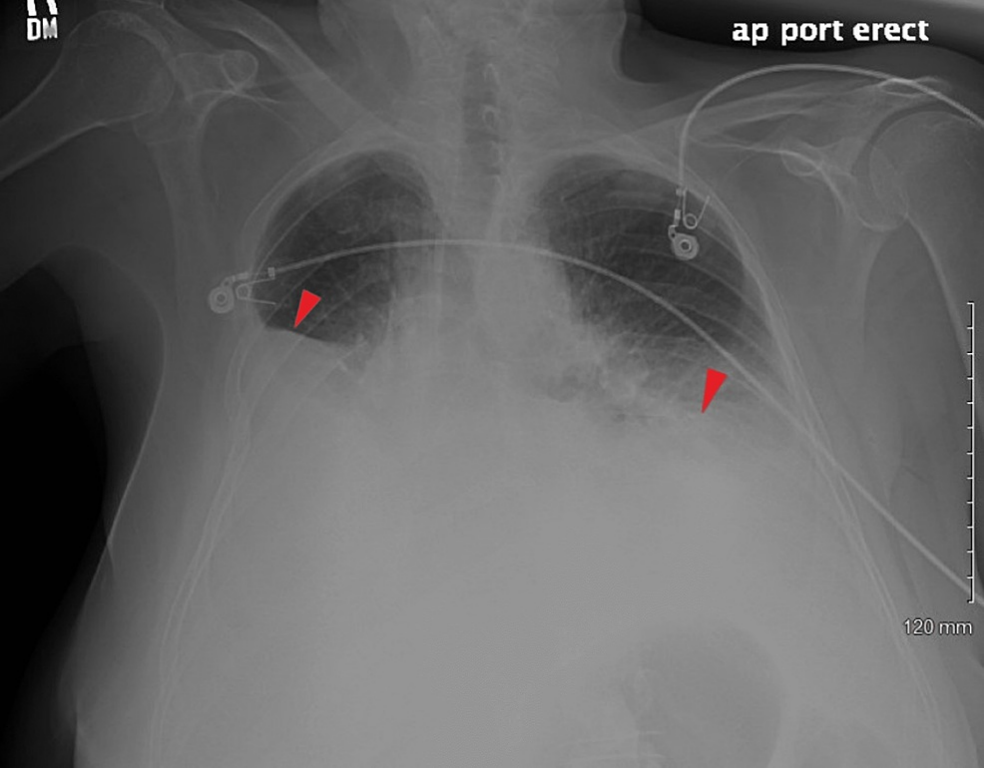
Chest X-ray showing large bilateral pleural effusions, more on the right side than the left, three days post thoracocentesis, and nine days post LNF. LNF: laparoscopic Nissen fundoplication
